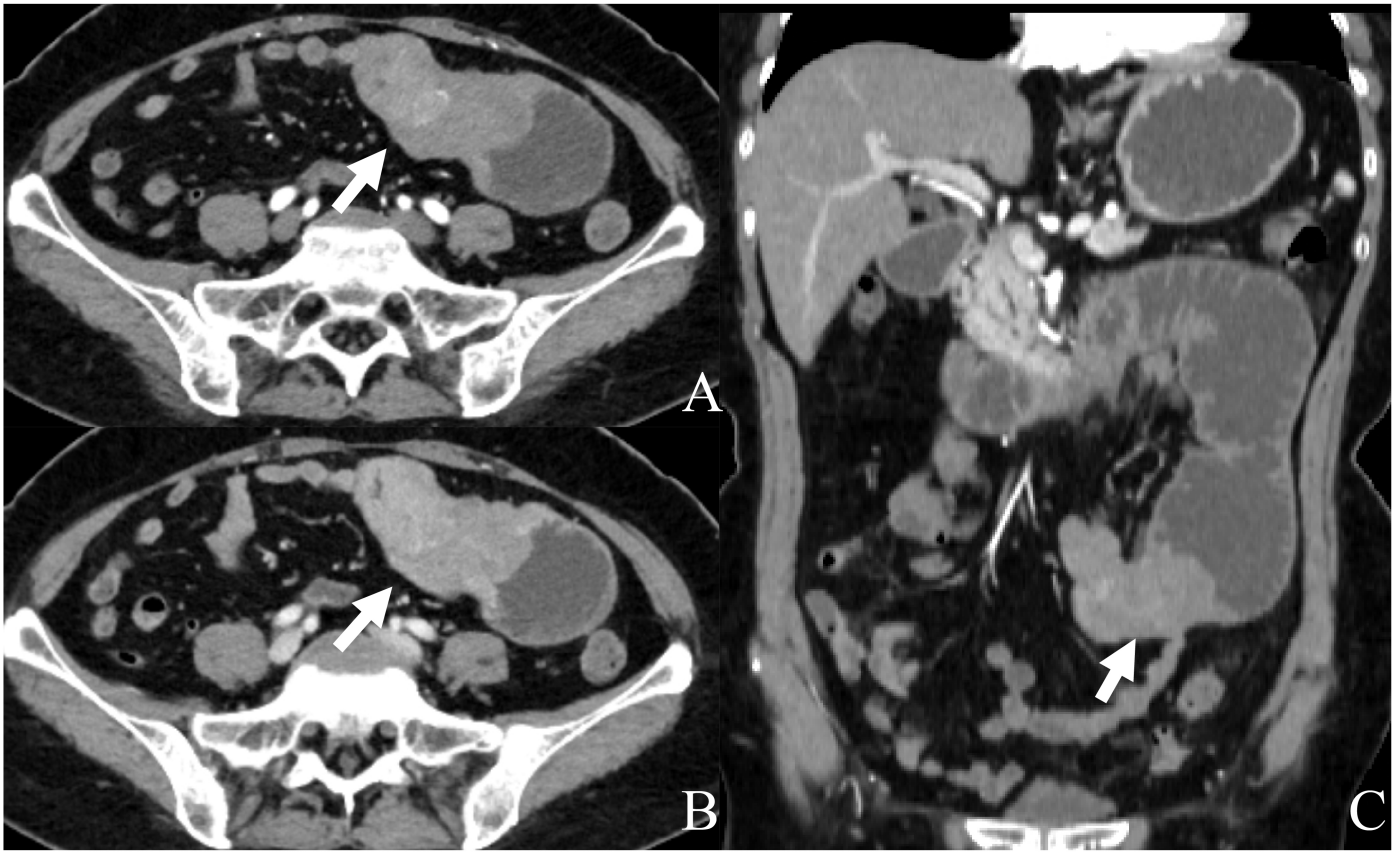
Abdominal CT with contrast: (A) axial arterial phase; (B) axial venous phase; (C) coronal arterial phase. Contrast-enhanced CT displays a well-defined mass of 7.8 cm × 4.2 cm × 5.1 cm (arrow) originating from the small bowel with heterogeneous enhancement, distal intestinal dilatation, and pneumatosis.
